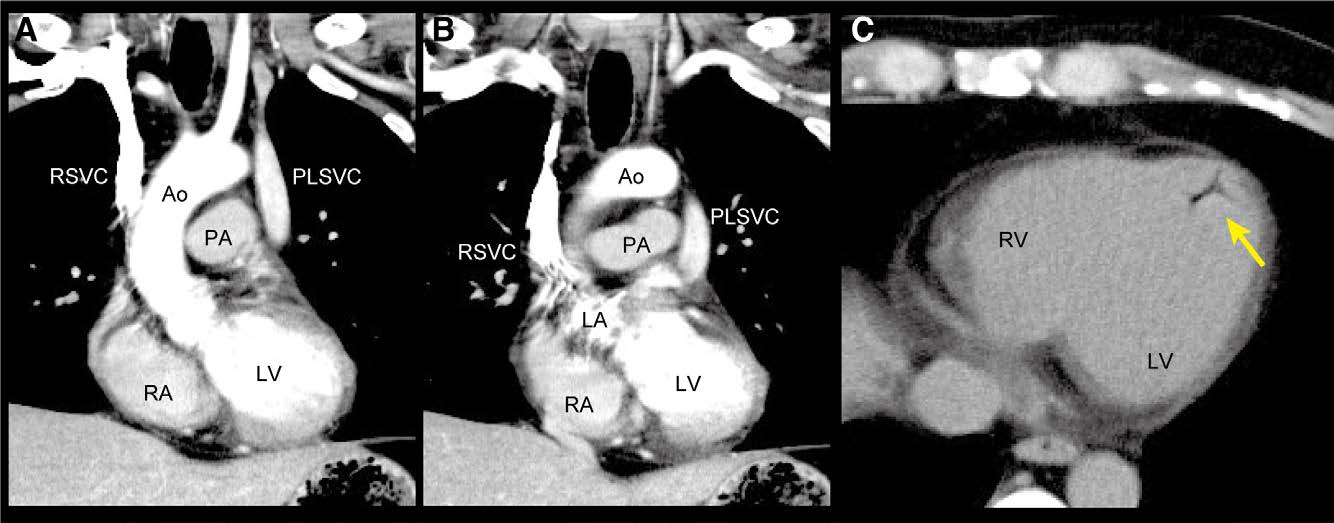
Cardiovascular CT angiography. (A, B) Coronal sections of contrast-enhanced images show the venous anomaly of the RSVC draining into the LA. (C) Transverse section of a noncontrast image shows air in the left ventricle, indicated by a yellow arrow. Ao = aorta, CT = computed tomography, LA = left atrium, LV = left ventricle, PA = pulmonary artery, PLSVC = persistent left superior vena cava, RA = right atrium, RSVC = right superior vena cava, RV = right ventricle.
